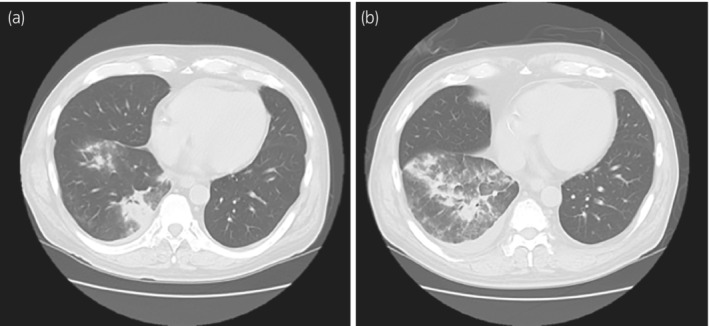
Thoracic CT (a) at disease onset and (b) 4 days after onset.
